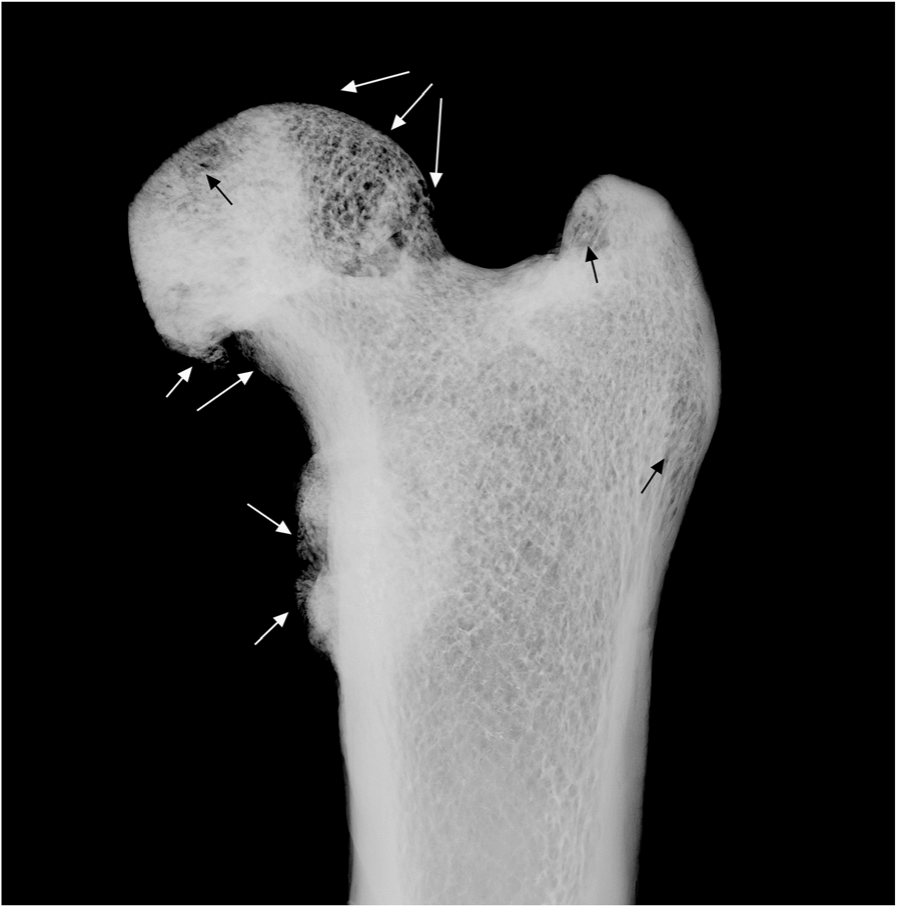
Radiograph of the right femur of *Tamandua tetradactyla* (NPM 069). White and black arrows, radiolucent regions indicating bone remodeling, associated with bone neoformation and bone rarefaction
